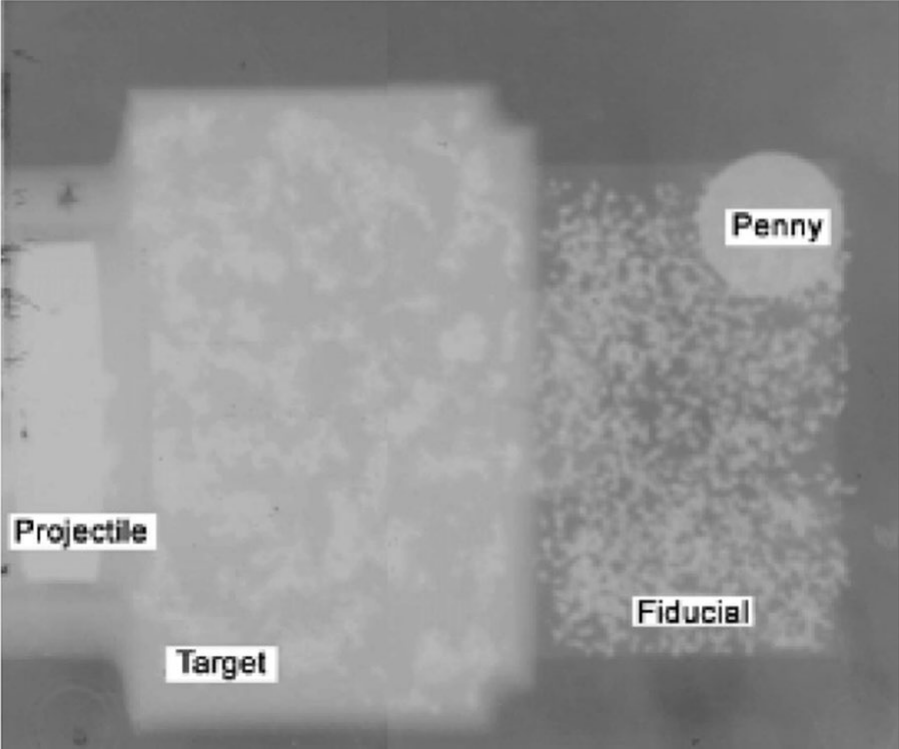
Flash X-ray of impact experiment. The UK 1 pence piece acts as a indicator of the field of view. The projectile traveling left to right and has struck the target, the fiducial markers do not move on the timescale of the experiment. [44]
